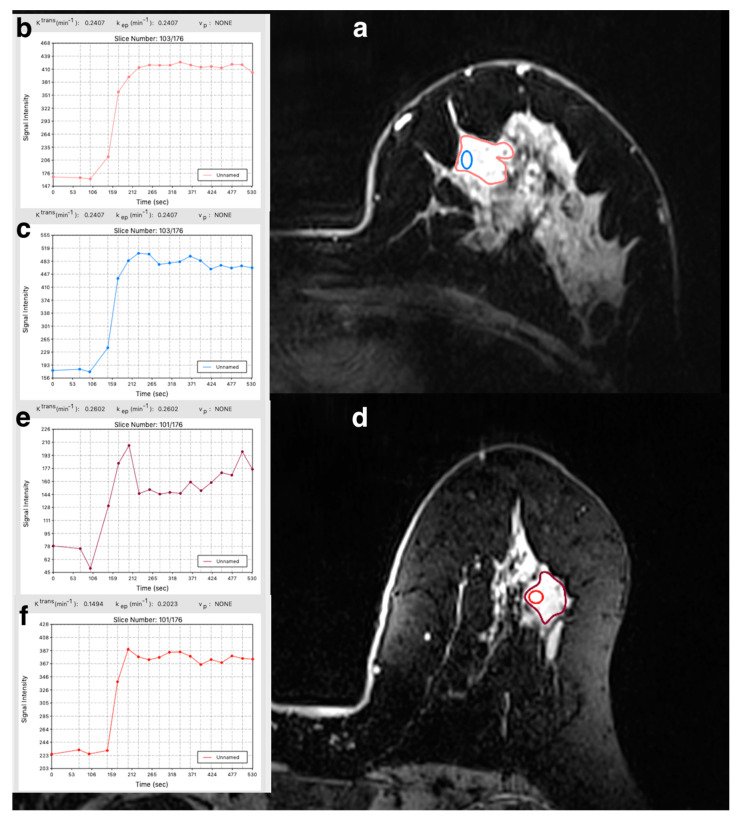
(a) A 74-year-old woman with invasive ductal carcinoma (IDC), luminal A, of the left breast on ultra-high-field dynamic contrast-enhanced magnetic resonance imaging at 7T. (b) Whole-tumor region of interest pharmacokinetic values and signal intensity curve. (c) Standard 10 mm^2^ ± 1 mm^2^ region of interest pharmacokinetic values and signal intensity curve. (d) A 66-year-old woman with invasive ductal carcinoma (IDC), triple-negative (TN), of the left breast on ultra-high-field dynamic contrast-enhanced magnetic resonance imaging at 7T. (e) Whole-tumor region of interest pharmacokinetic values and signal intensity curve. (f) Standard 10 mm^2^ ± 1 mm^2^ region of interest pharmacokinetic values and signal intensity curve. Note: ROI: unnamed = no specific name was assigned to the ROI. Graph inserts are also provided separately as supplemental figures.
